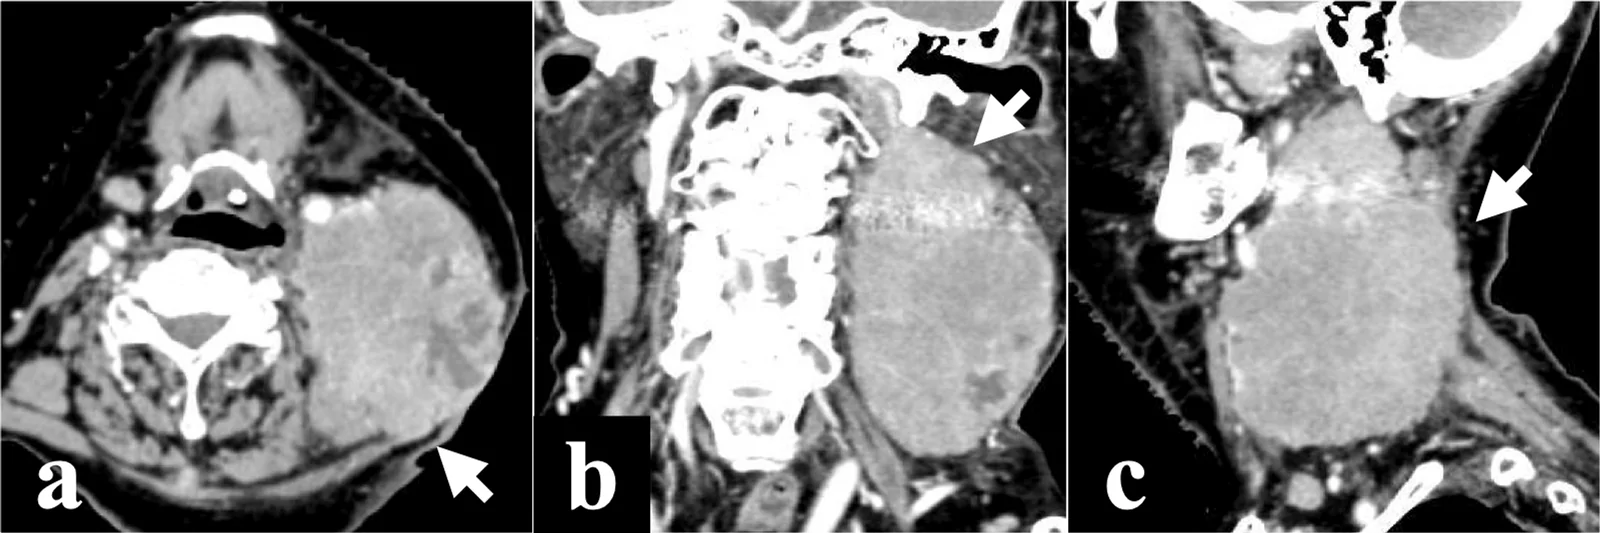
Contrast-enhanced CT images of the patient's neck Contrast-enhanced CT demonstrating the left cervical lymph node metastasis (measuring 10 × 8 cm) before SBRT (white arrow). (a) Axial, (b) coronal, and (c) sagittal views. CT; computed tomography, SBRT; stereotactic body radiotherapy.
